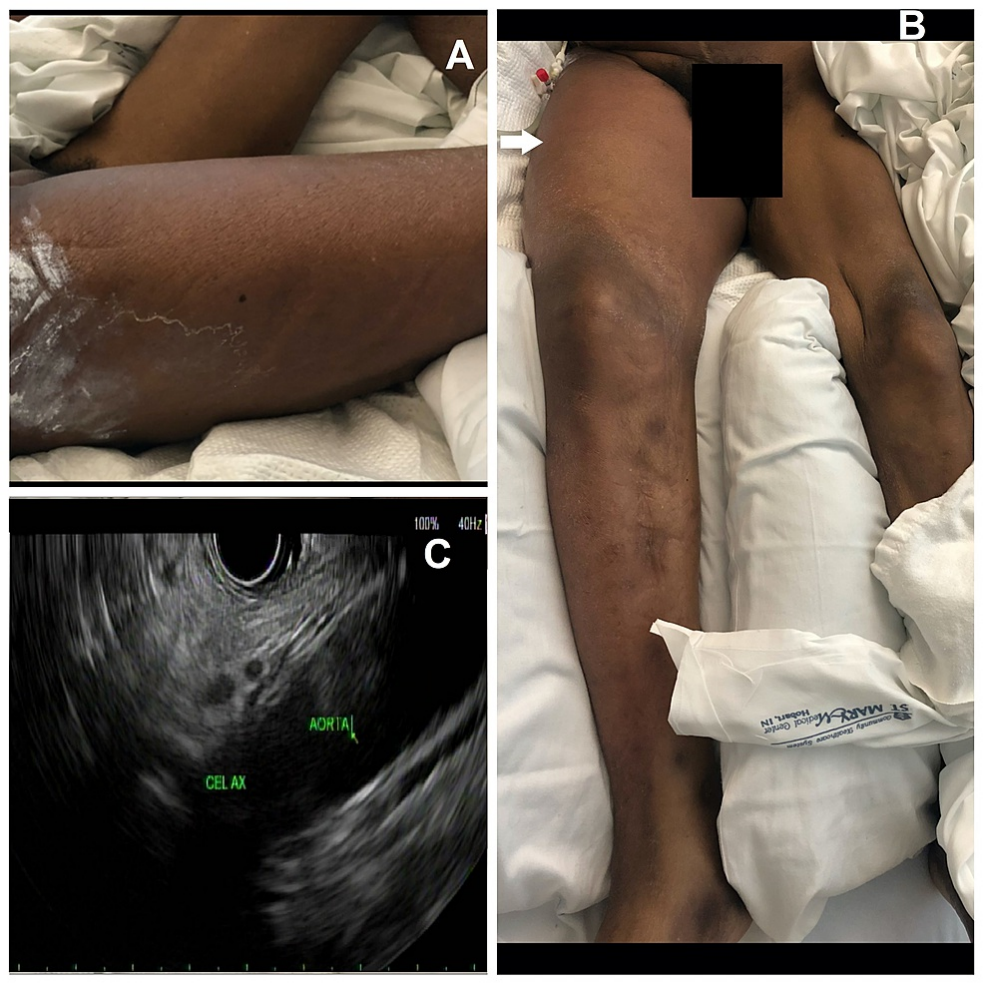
A and B: Images showing right thigh and gluteal region swelling with associated erythema. C: EUS showing diffusely hypoechoic pancreas with lobularity and multiple linear and focal areas of echogenicity suggestive of the atrophic pancreas with calcifications. EUS, endoscopic ultrasound.
